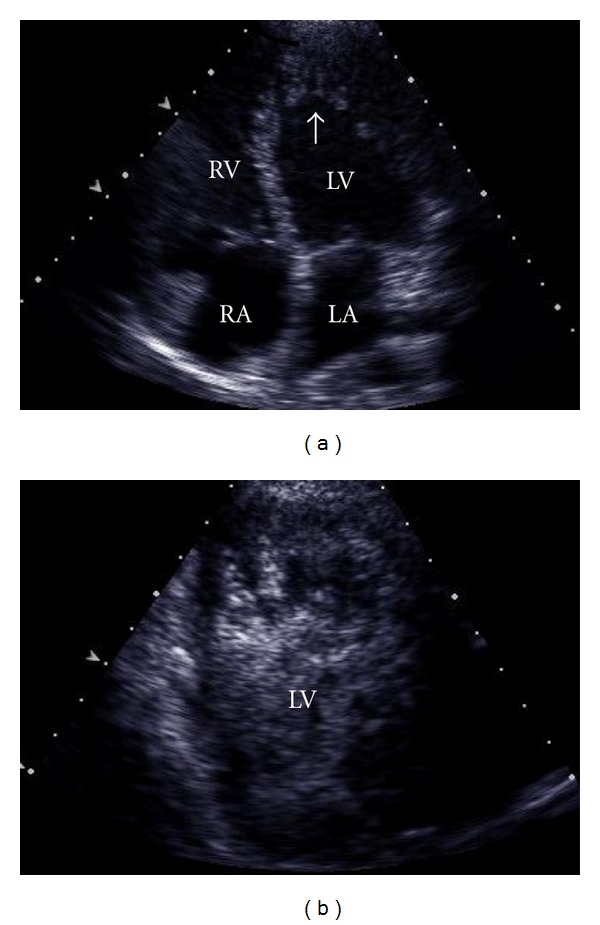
Echocardiogram of the heart. (a) Apical view demonstrating marked trabeculation of the left ventricular apex (white arrow). (b) It shows contrast agent visualized between the ventricular trabeculations. RA: right atrium; LA: left atrium; LV: left ventricle; RV: right ventricle.
